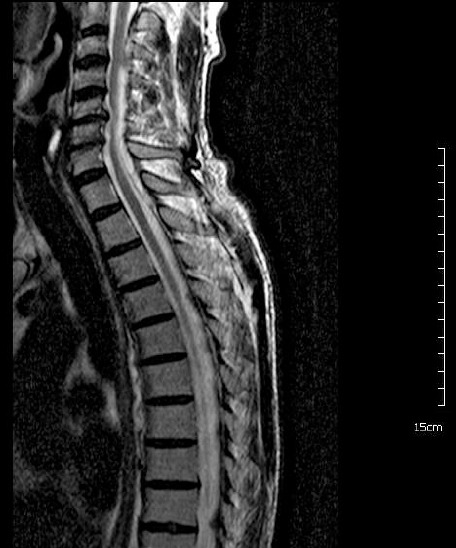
A cystic mass lesion which was extends between C3 to T2 in preoperative T2 MRI sagittal section*.
